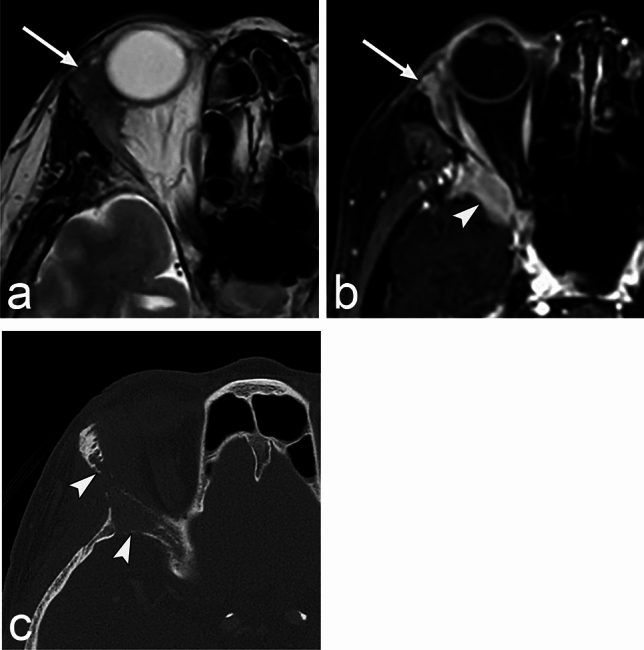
Adenoid cystic carcinoma of the lacrimal gland of a woman in her 70s with pain in the right eye. a, b MRI shows an ill-defined enhanced mass (arrow) in the right lacrimal gland. The mass shows iso-signal intensity compared to the brain cortex on the T2 weighted image. The mass invades the sphenoid bone (arrowhead). c CT shows bone destruction in the zygomatic and sphenoid bones (arrowheads)
